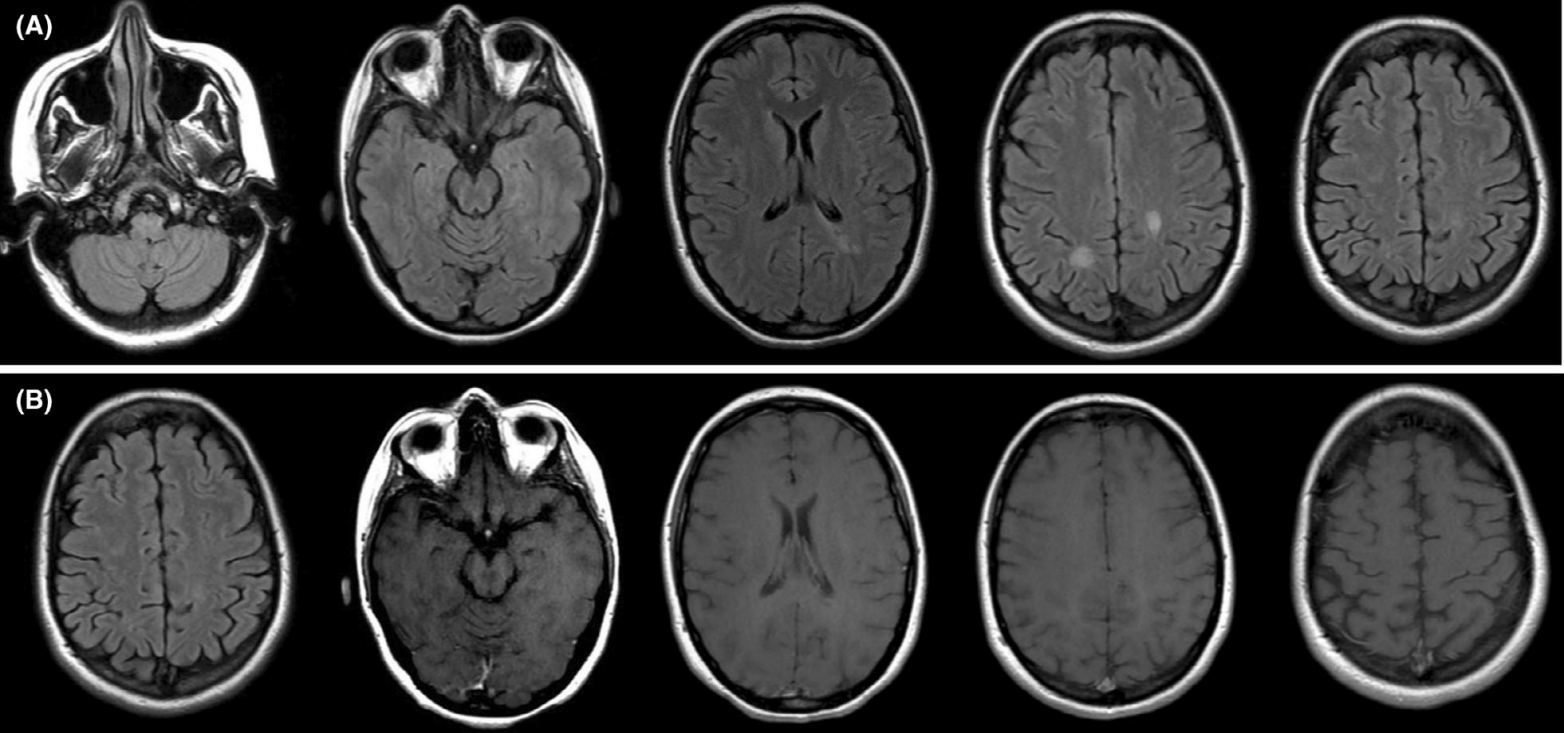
MRI brain with and without contrast 4 months after admission: (A) T2 FLAIR sequence showing improved hyperintensities in the medulla and periventricular white matter. (B) T1 post‐contrast sequence showing resolution of enhancing lesions. No new lesions seen.
